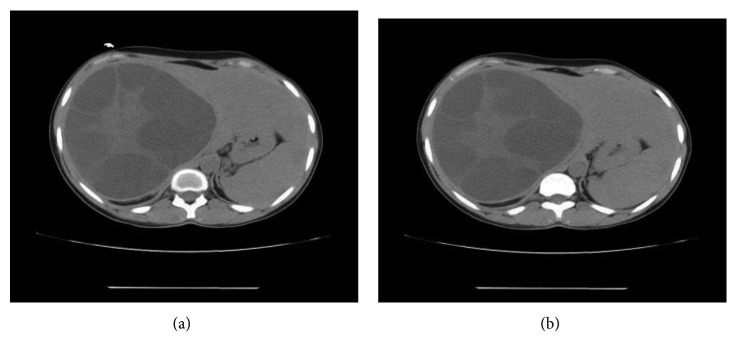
Noncontrast CT scan showing a giant cyst of the liver.
